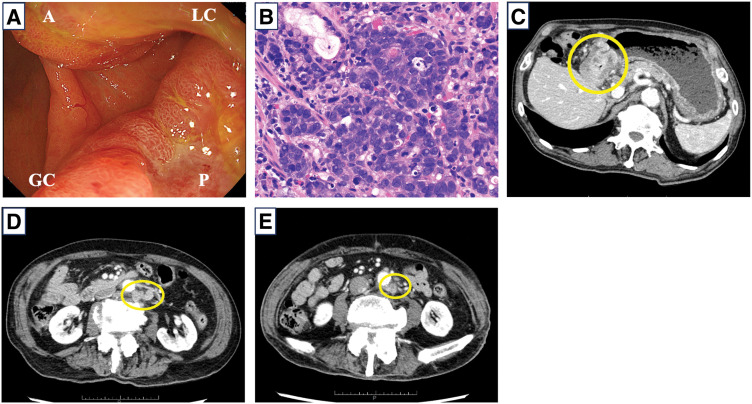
Upper gastrointestinal endoscopic, pathological findings, and contrast-enhanced CT before chemotherapy. (A) Upper gastrointestinal endoscopy showing circumferential stenosis in the lower gastric body due to a type 3 tumor. (B) A biopsy revealed differentiated adenocarcinoma (por1). (C) Contrast-enhanced CT showing circumferential wall thickening at the tumor site. (D, E) Para-aortic lymph nodes nos. 16b1 (D) and 16b2 (E) were enlarged (circles). A, anterior wall; GC, greater curvature; LC, lesser curvature; P, posterior wall
